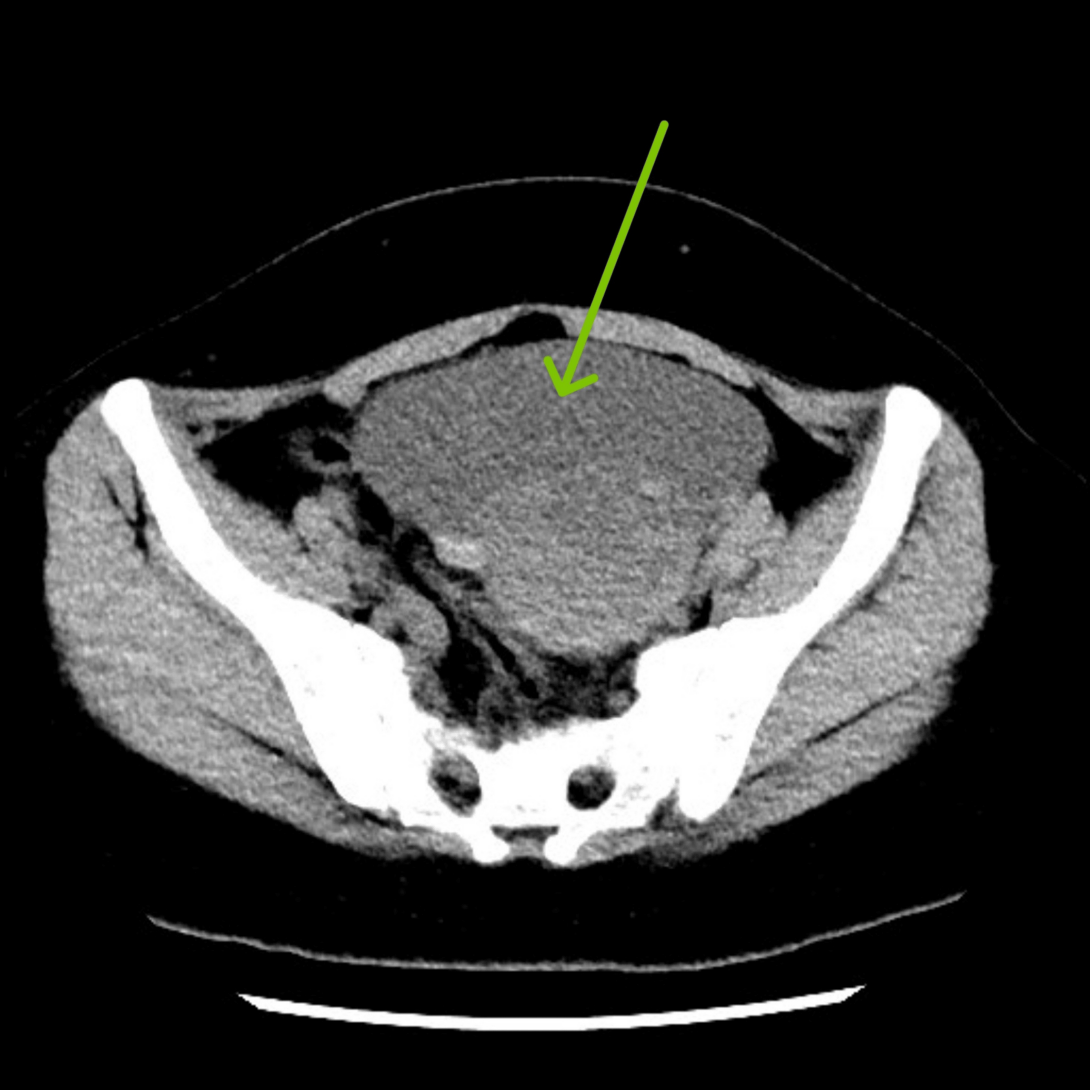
CT abdomen and pelvis, axial view. The arrow points to the left adnexal cyst in the axial view.
